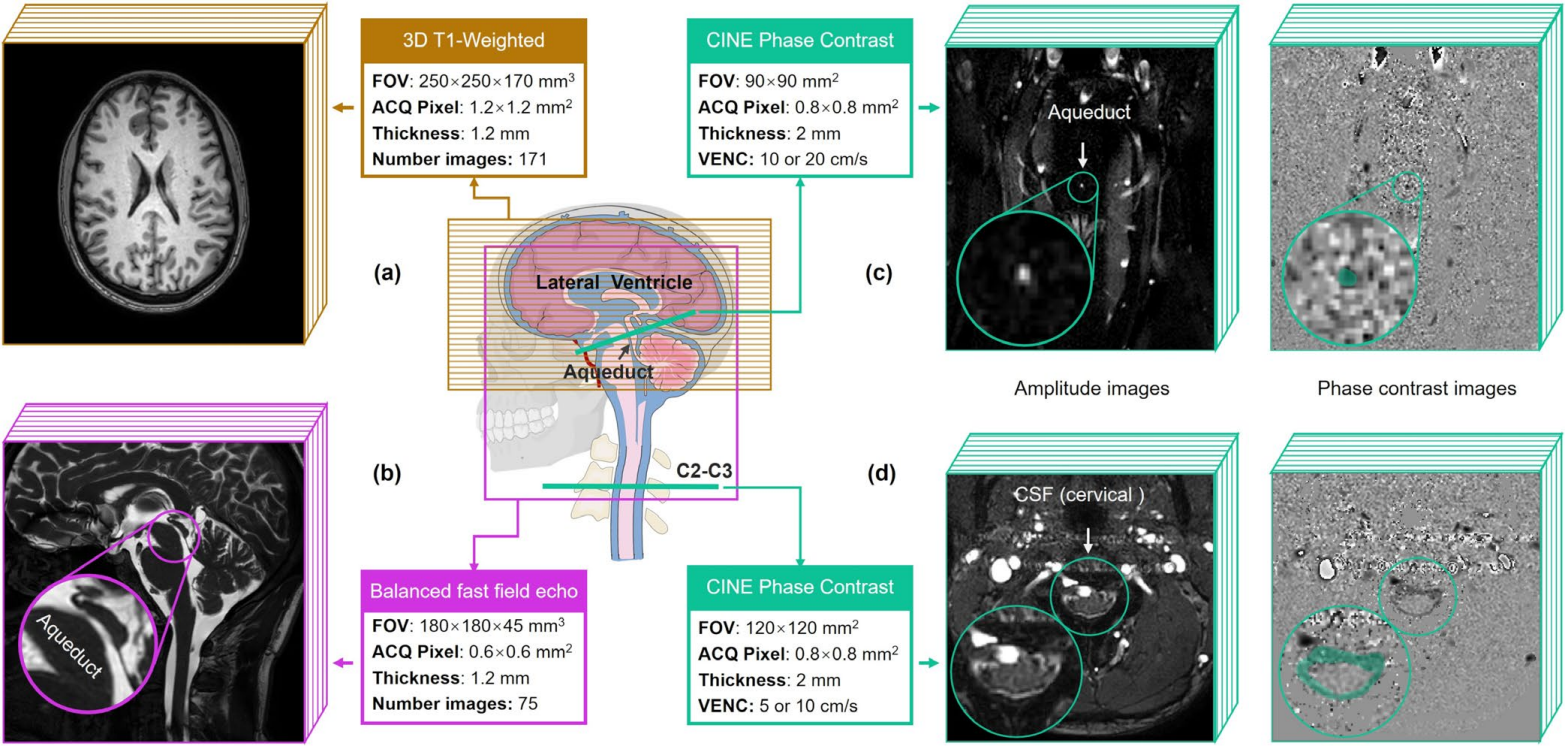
Examples of the three MRI acquisition sequences. A transverse 3D T1‐weighted sequence (a) covering the entire brain was used to calculate ventricular and brain areas. A sagittal Balanced Fast Field Echo sequence (b) was employed to calculate aqueductal resistance. Two CINE Phase Contrast sequences were used to calculate CSF flow at the aqueduct (c) and C2–C3 (d) level. ACQ, acquisition; FOV, field of view; VENC, velocity encoding.
